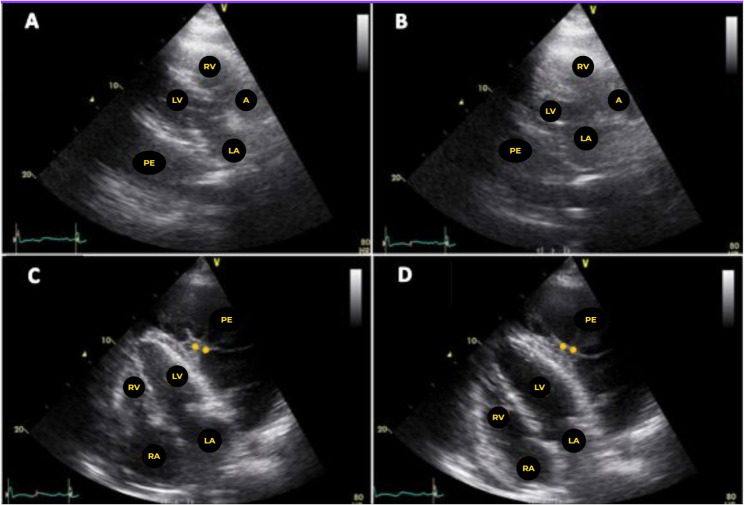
Transthoracic echocardiogram. A and B: Parasternal long-axis views demonstrating extensive circumferential pericardial effusion (DP) without evident diastolic compression of the right ventricular free wall. C: Four-chamber apical view in systole showing the magnitude of the pericardial effusion (DP). D: Four-chamber apical view in diastole highlighting the presence of fibrin strands (Fibrin) within the effusion, suggesting an exudative or malignant process. RV = Right Ventricle, LV = Left Ventricle, RA = Right Atrium, LA = Left Atrium, PE = Pericardial Effusion A = Aorta Fibrin strands
